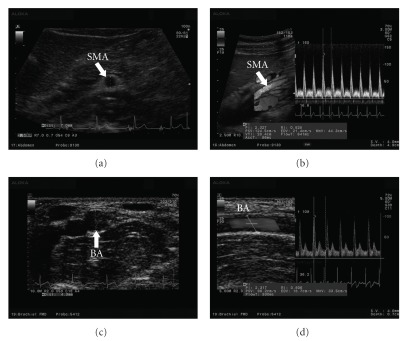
Hemodynamic data obtained by ultrasound. (a) VD of the SMA, (b) blood flow velocity of the SMA, (c) vessel diameter of the BA and (d) blood flow velocity of the BA.
